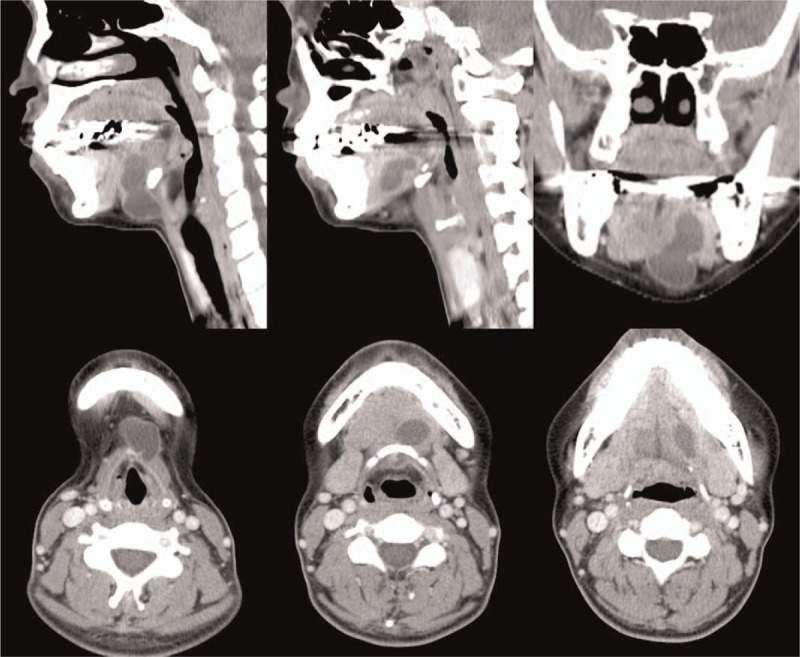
CT scan showing a well-defined thin walled cystic lesion measuring approximately 3 cm in the midline infrahyoid region extending into the hyoid bone, reaching to the left mouth floor, between the genioglossus muscle and mylohyoid muscle. The thyroid gland is within the normal position (not shown).
